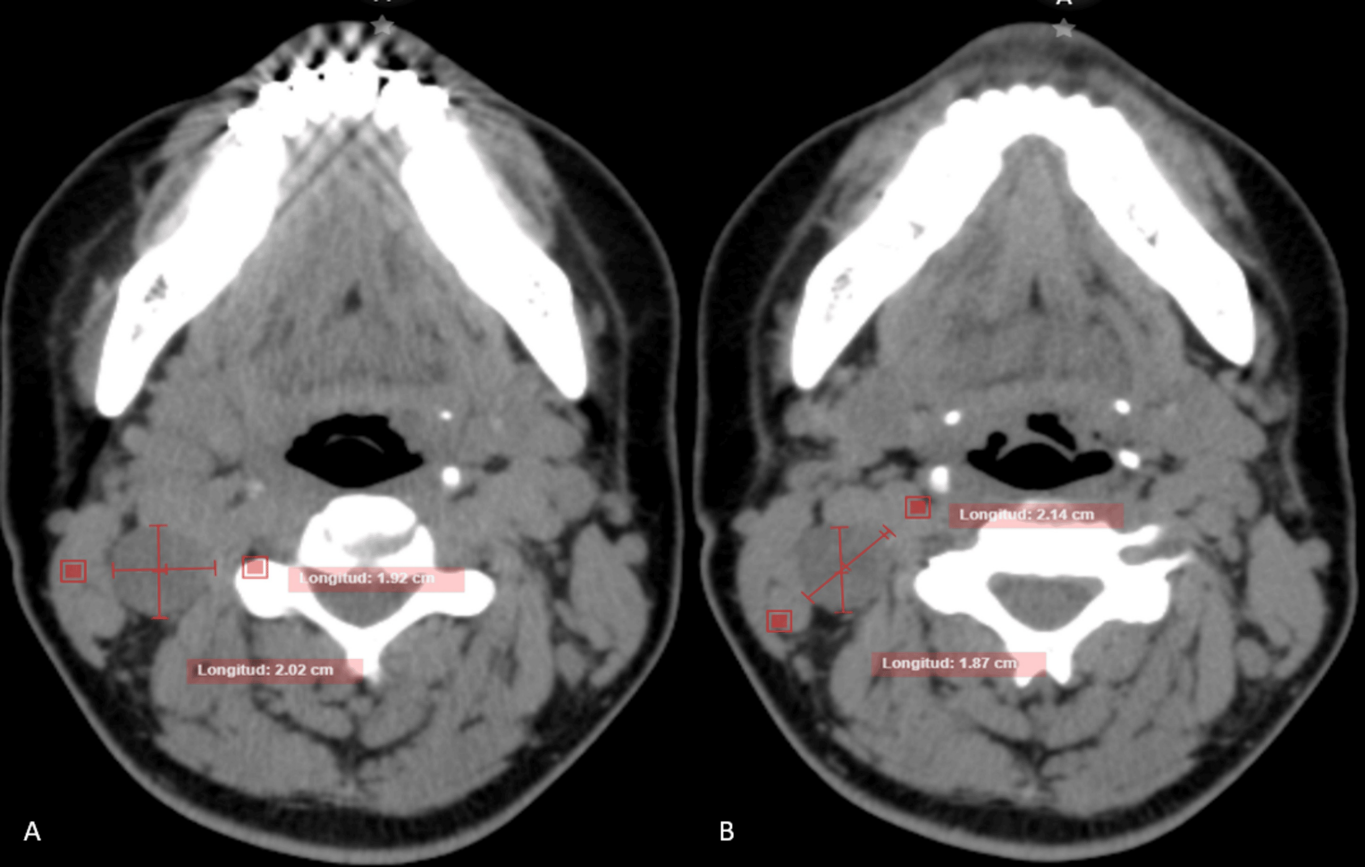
Axial CT slices of the head and neck shows a homogeneous, ovoid-shaped parapharyngeal mass. A) Measures 1.92x2.02 cm B) Measures 2.14x1.87 cm
